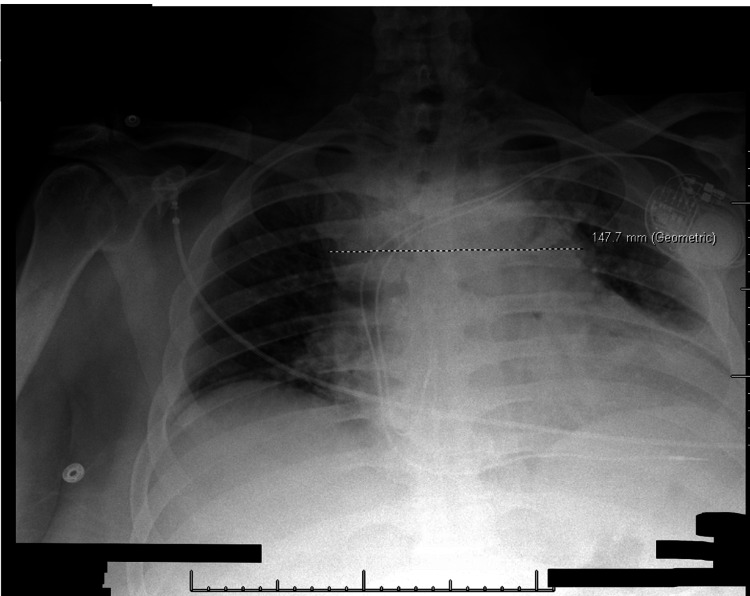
Chest 1 View AP/PA X-ray A frontal view of the chest was obtained. There is a stable appearance of a left chest pacemaker. The heart is mild to moderately enlarged with enlargement of the mediastinum measuring approximately 15 cm. There is mild pulmonary vascular congestion. Mild linear densities are seen at the bilateral lung bases which may represent atelectasis.
